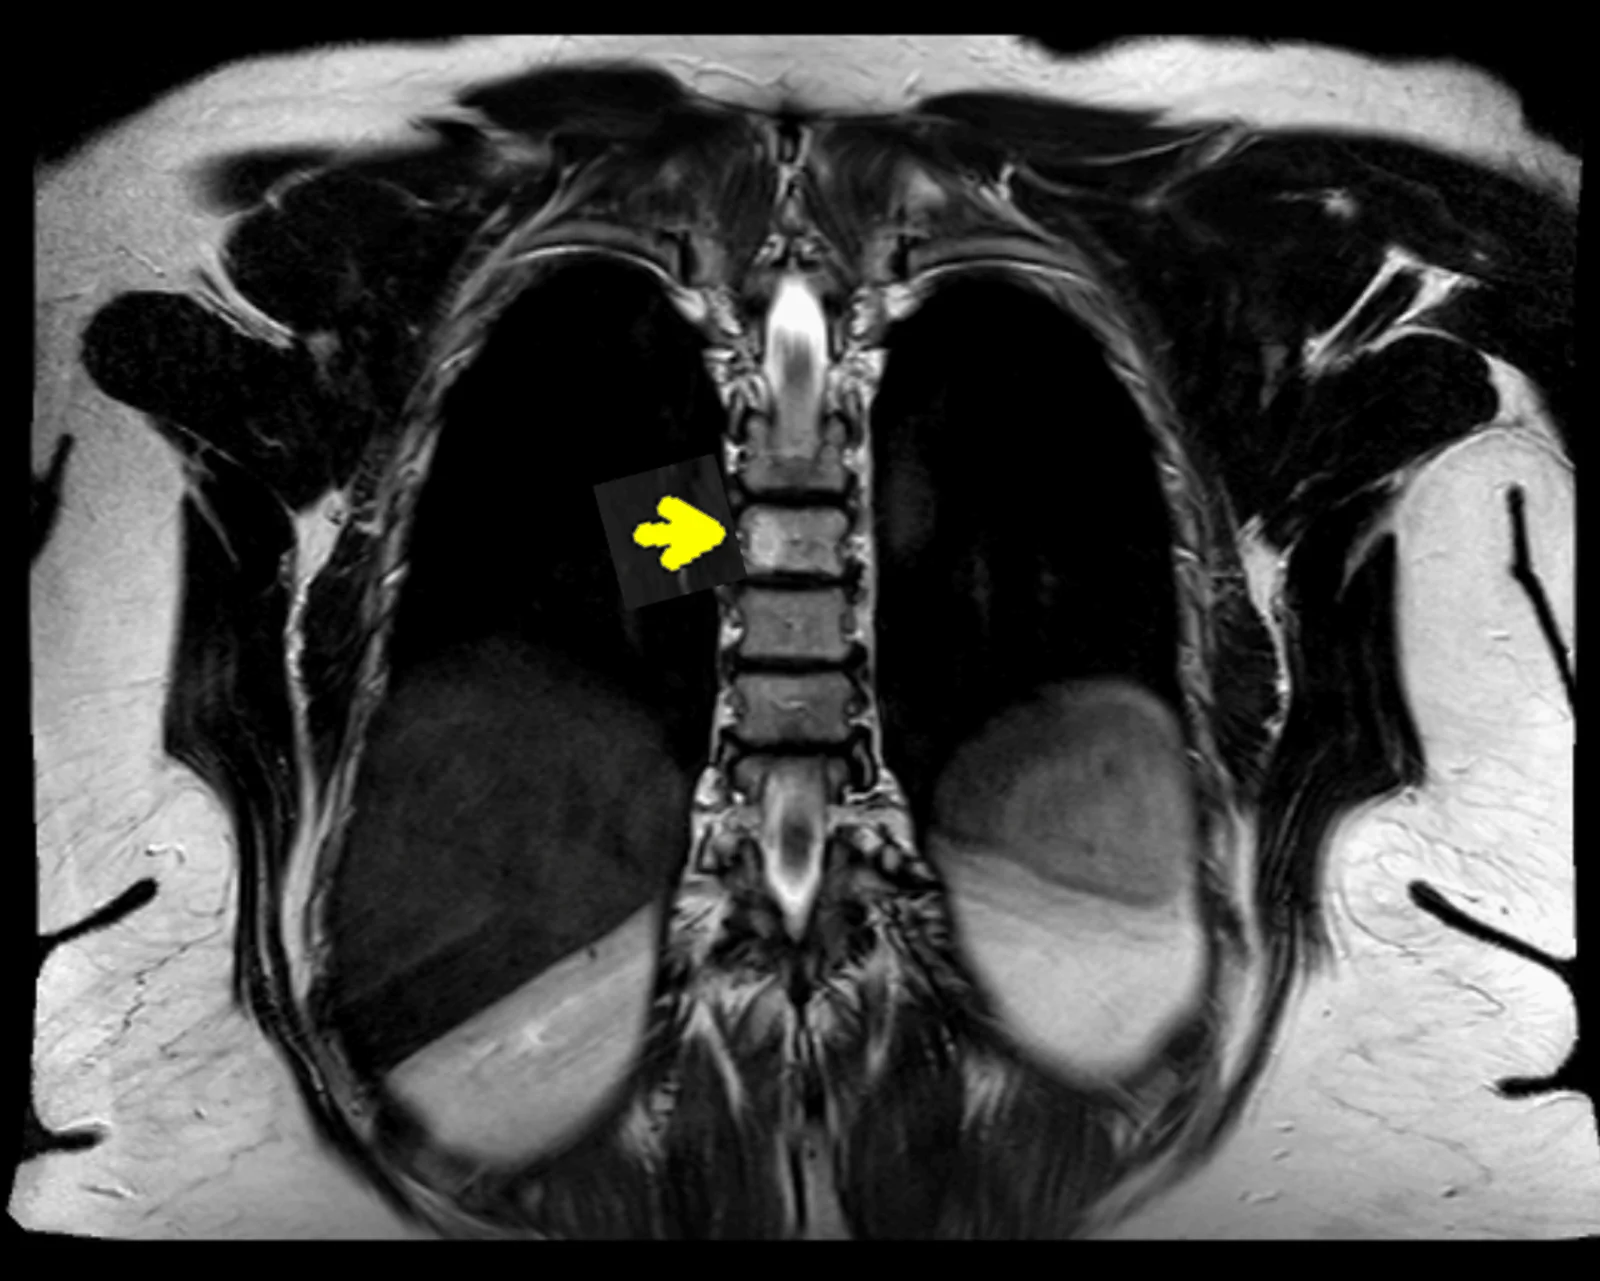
MRI of the thoracic spine (coronal view). Coronal view of MRI of the thoracic spine with and without contrast showing linear T2 hyperintensity in the thoracic spinal cord at T7-8. The signal abnormality is slightly more eccentric to the left. Demyelinating disease would be in the differential.
